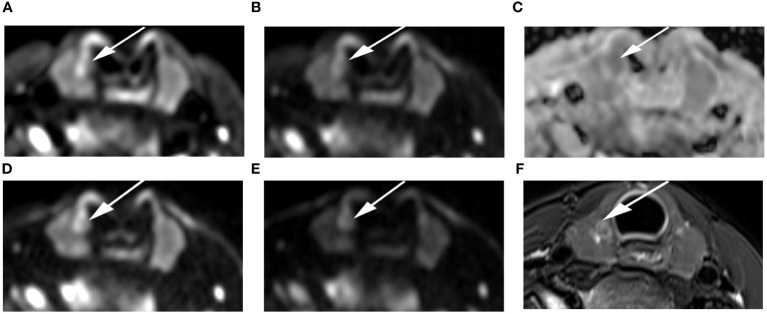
Images of a 24-year-old female with right lobe micropapillary carcinoma (arrow): DWI image with a b-value of 500 s/mm^2^
